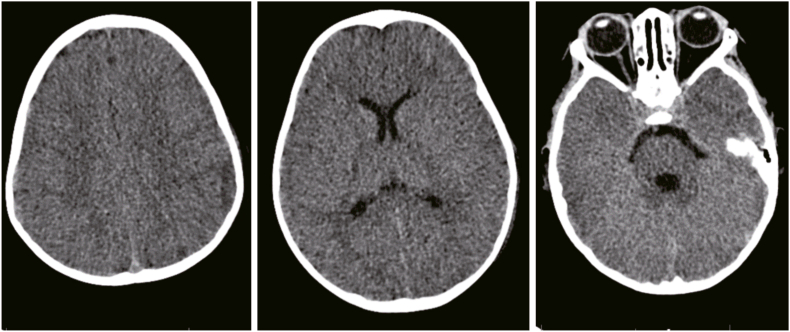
Initial axial uncontrasted CT of the brain showing left parietal hypodensity underlying the clinical area of the full-thickness scalp burn wound.
